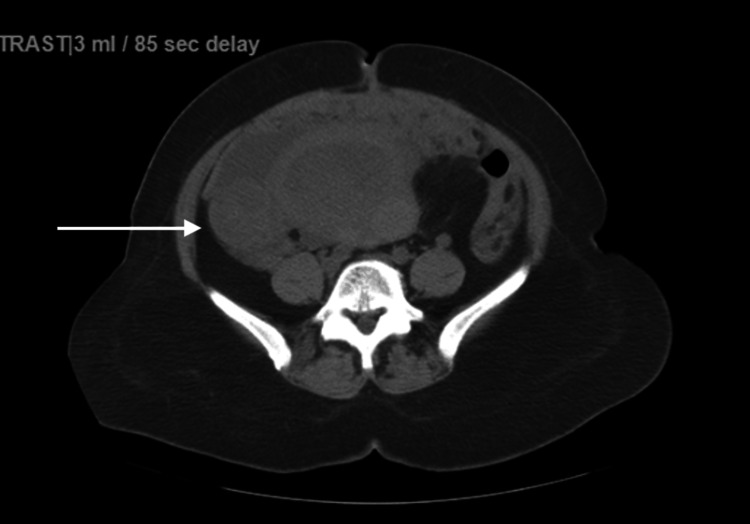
Abdominal computed tomography scan without enhanced contrast shows an enlarged uterus with a right adnexal mass measuring up 4.6 cm.
